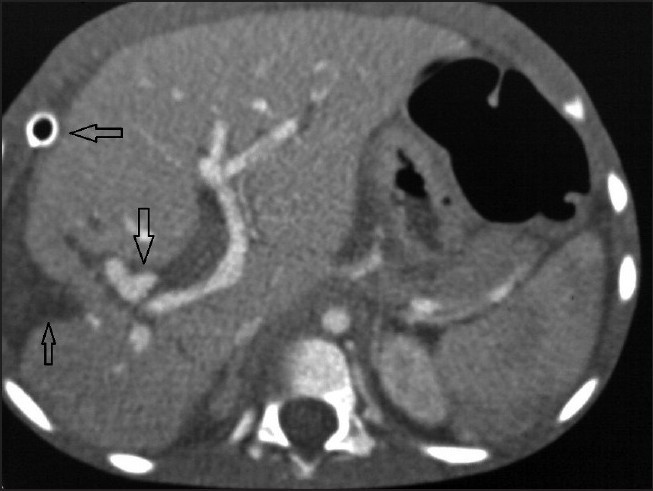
Axial contrast-enhanced CT image showing defect in the right lobe of the liver with adjacent perihepatic fluid collection (upward arrow). Enhancing lesion is seen anterior to the right branch of the portal vein (down ward arrow). Drain tip is seen anteriorly, in perihepatic location (leftward arrow)
